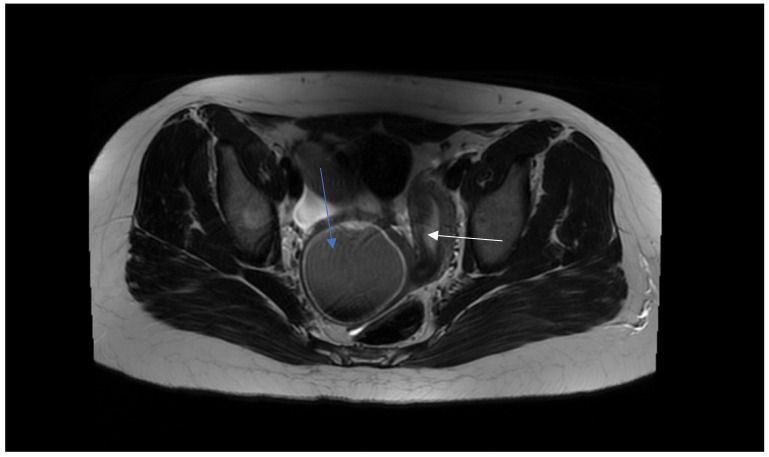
MRI presents hematocolpos (blue arrow) and a left healthy uterus (white arrow).
